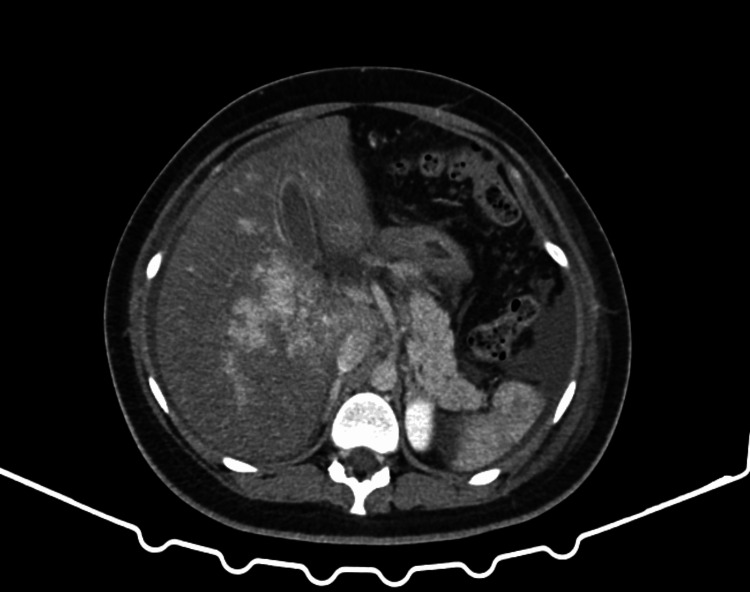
CT scan Hepatomegaly with hypertrophy of segment I and diffuse thrombosis of hepatic veins
